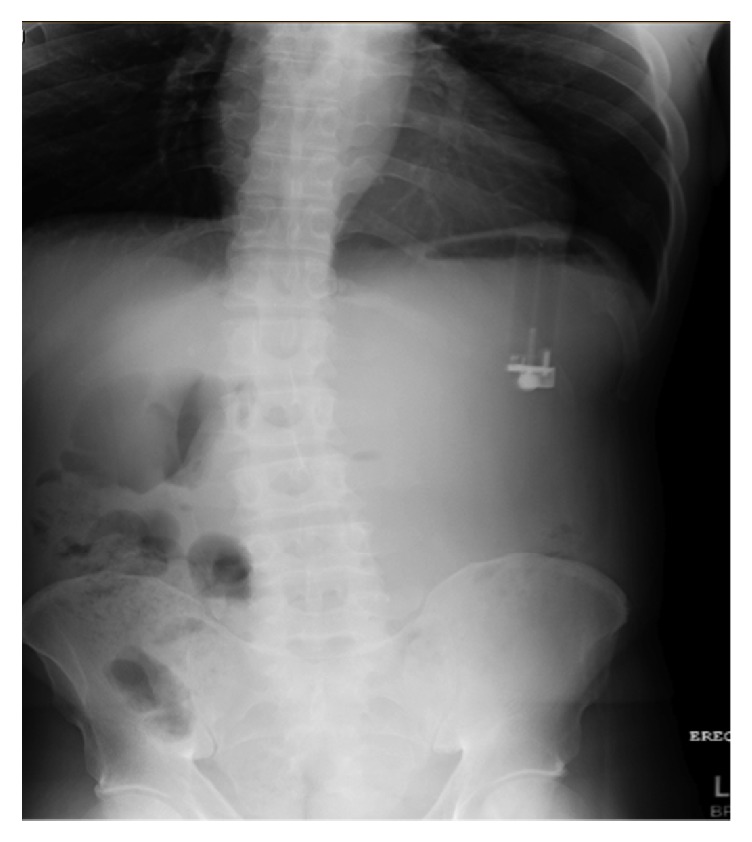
Cigarette lighter on abdominal X-ray.
